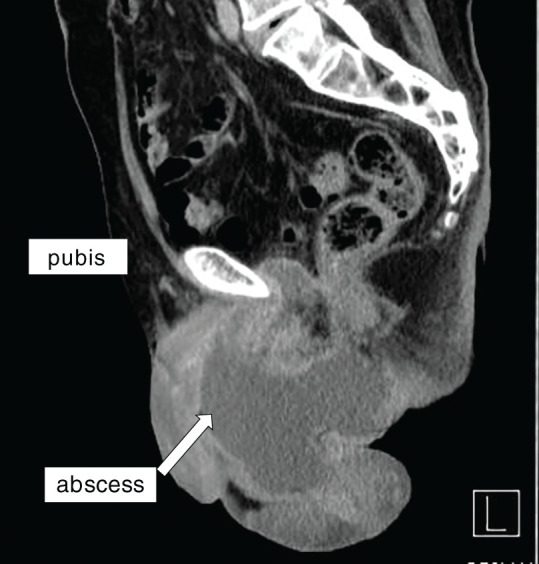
In Case 2, the patient presented with perineal swelling. CT revealed abscess formation, which was treated with incision and drainage.
